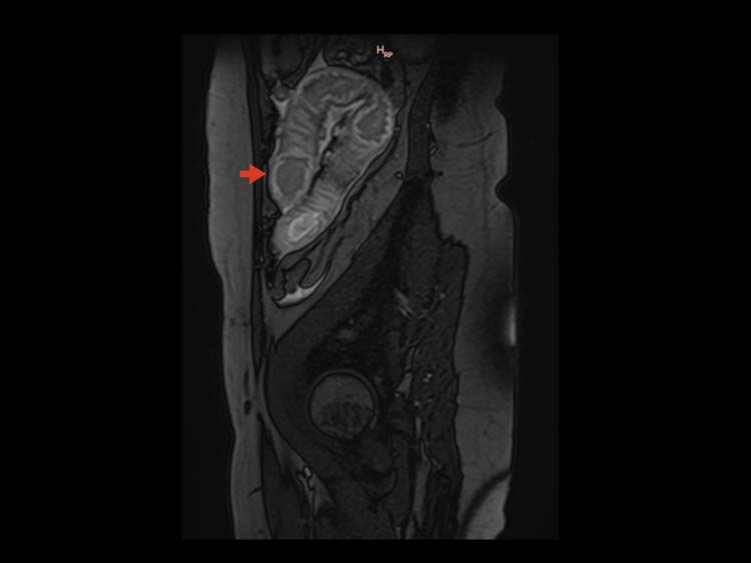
Sagittal section on MRI showing parietal thickening of the intestinal wall. Arrow: thickened intestinal wall.
